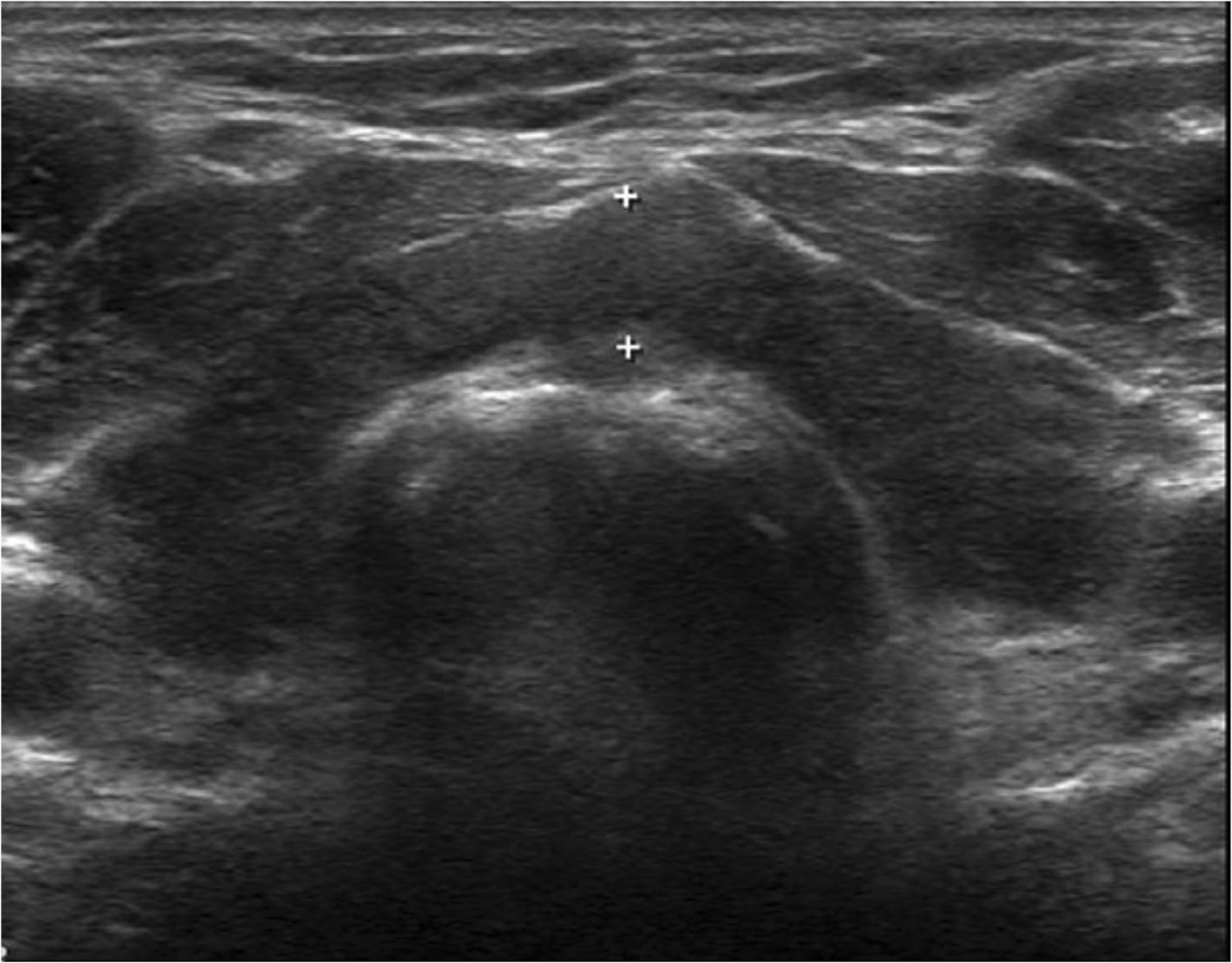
Transverse view of thyroid sonography revealed marked heterogenous echotexture of both thyroid glands
